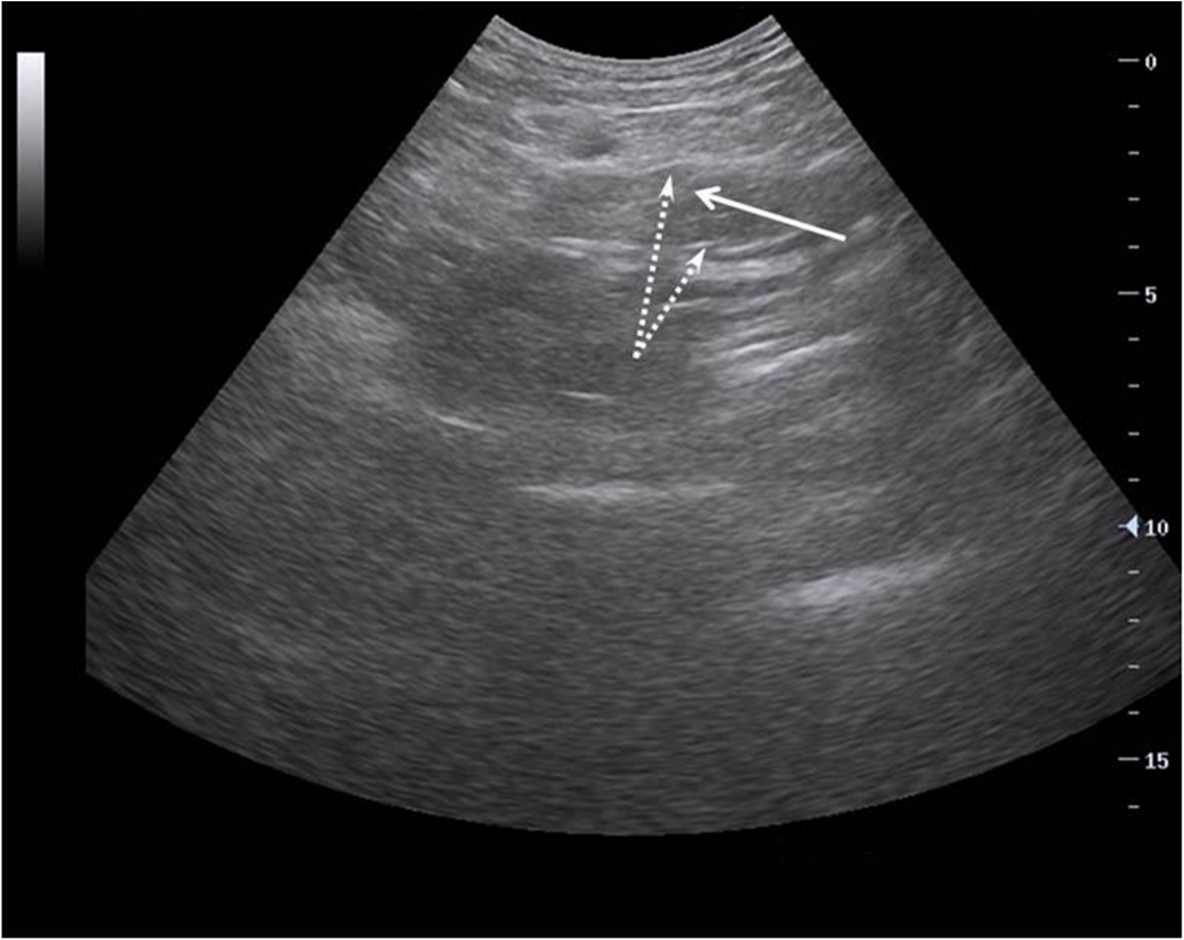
Normal transabdominal intestinal ultrasonography. This is a 36-year-old male. The intestine was screened with a curvilinear probe (5 MHz). The image shows normal intestinal diameter (< 2 cm), normal intestinal thickness (< 2 mm, dotted arrow), and normal folds (long without edema, solid arrow)
